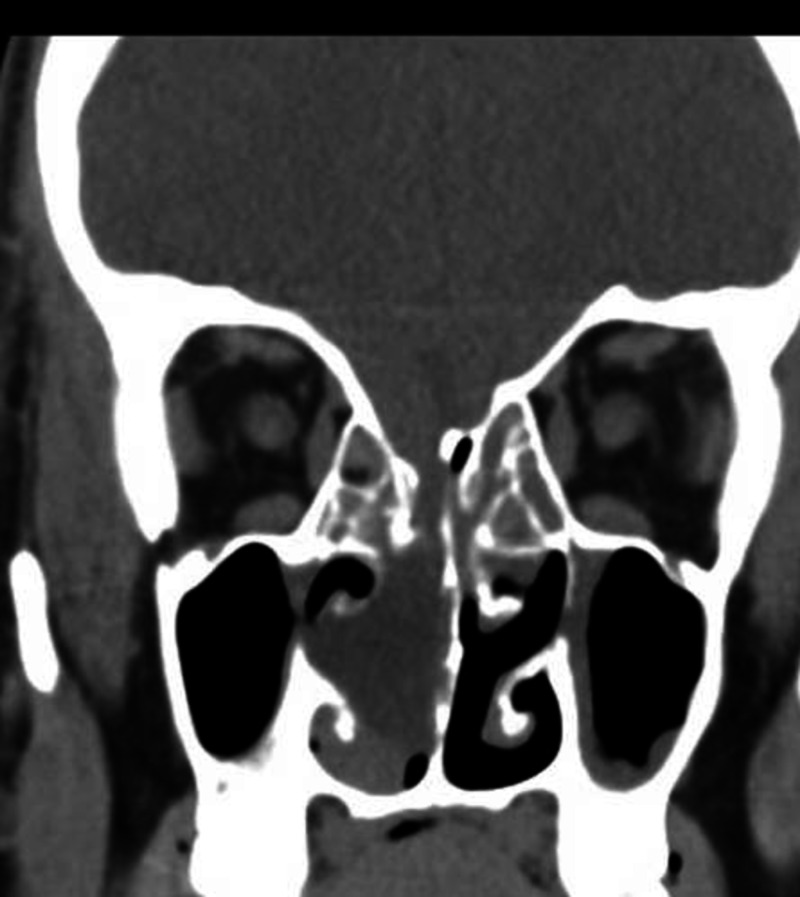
Coronal CT sinuses showing a right cribriform plate of ethmoid defect with soft tissue mass through defect. CT: computed tomography.
